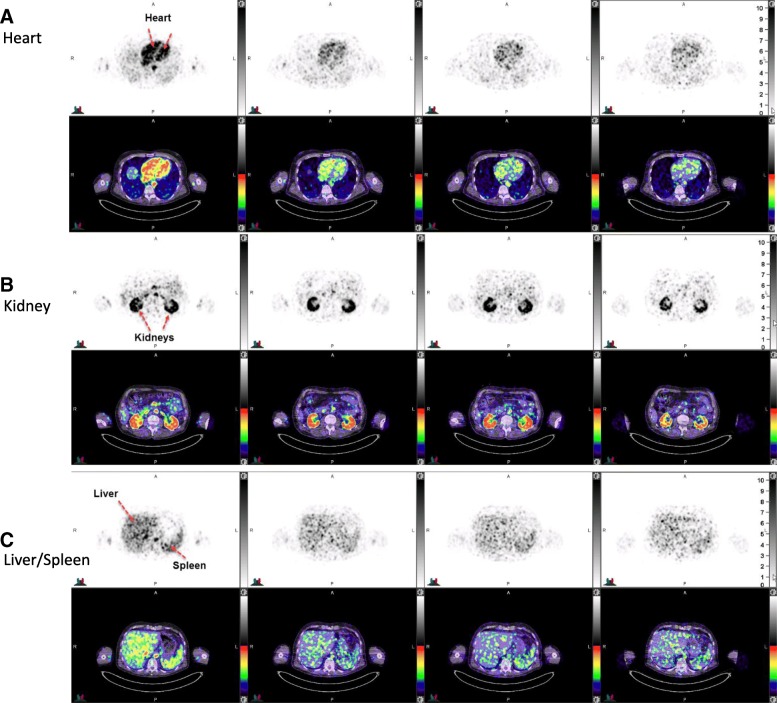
Transaxial images (top PET, bottom PET/CT fused images) across the a heart, b kidneys, and c liver/spleen from the same subject 135 presented in Fig. 1. Times post dose 7, 79, 120, and 168 h post dose. Additional transaxial images for additional subjects 105 and 127 are provided in the Additional file 1: Figures S3 and S5
